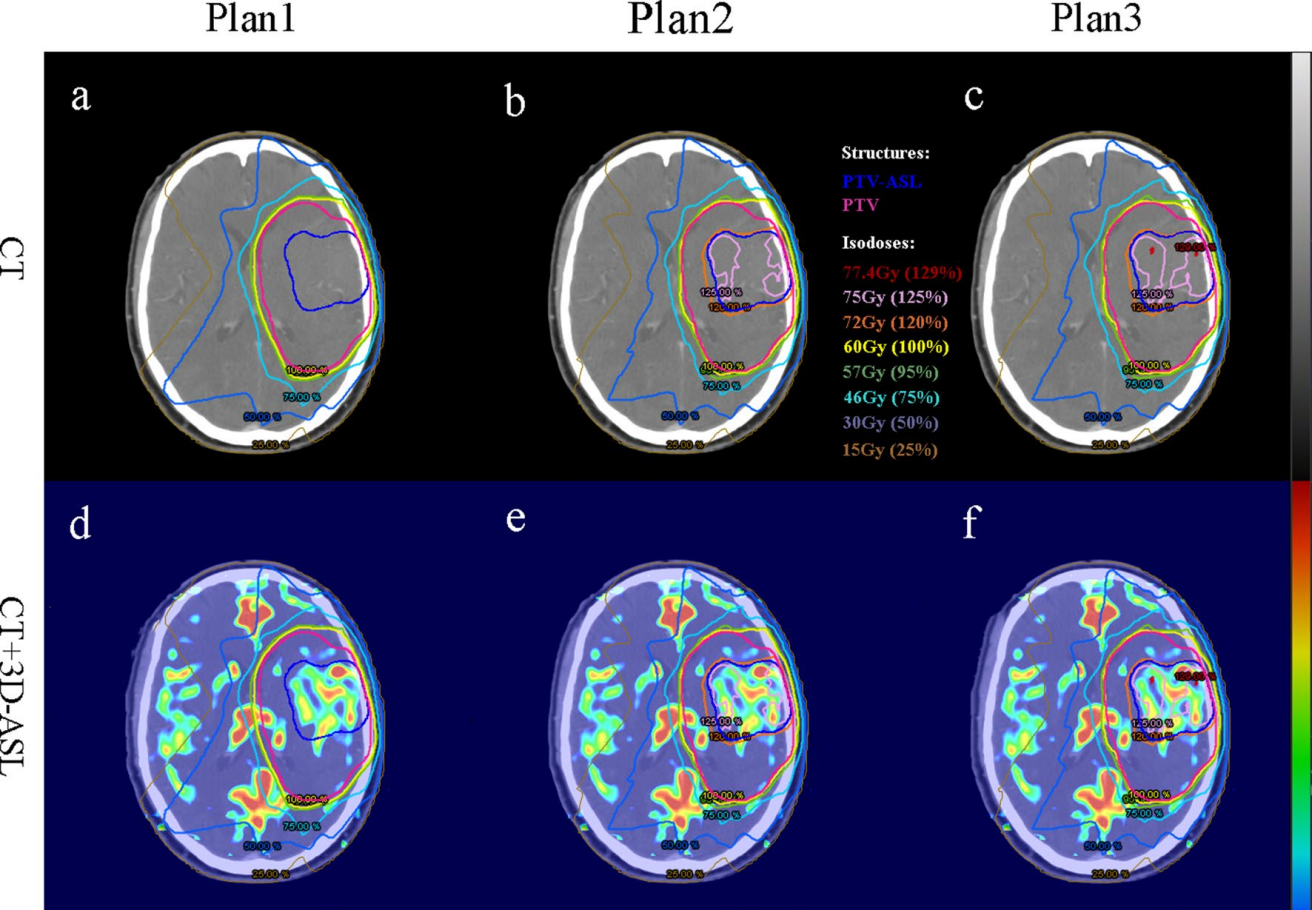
Isodose lines are shown for conventional plan (plan 1) and dose-painted plans (plan 2 and plan 3). Plan 1 (a and d): prescribed a homogeneous dose of 60 Gy to the PTV; plan 2 (b and e) and plan3 (c and f): the dose of PTV-ASL increased by 20% (equivalent to 72 Gy) based on plan 1, without the maximum dose constraint in plan 3. In a, b, c dose distribution is projected on the computed tomography (CT) images while in d, e, f dose distribution is projected on the three-dimensional arterial spin labeling (3D-ASL) images
